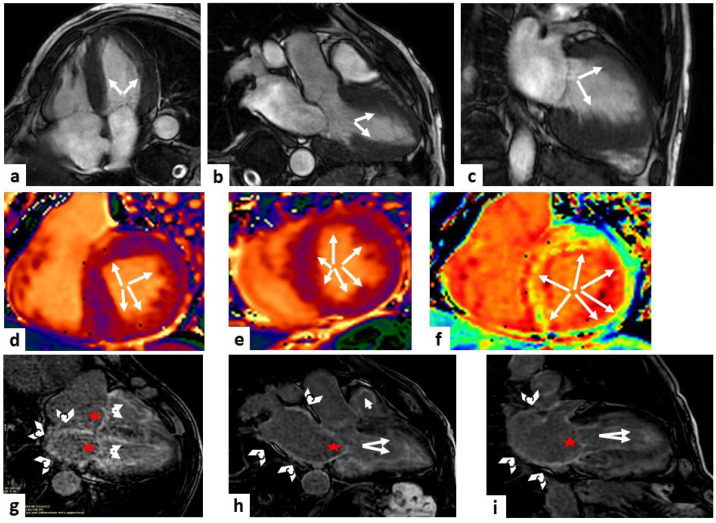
Cardiac amyloidosis. A case of a 79-year-old man, with magnetic resonance imaging (MRI) showing a hypertrophic (white arrows) left ventricle (LV) of 17 mm in the steady-state free precession (SSFP) sequences (panels (a–c) show four-chamber, three-chamber, and two-chamber views, respectively). Mid panels show diffuse high values at T1 mapping sequences, represented by the orange tonality as indicated by the white arrows (panels (d,e) at the level of the basal and mid-LV short-axis views, respectively) and diffuse high values at extracellular volume (ECV) sequence, represented by the yellow tonality as indicated by the white arrows (panel (f) at the level of the basal LV short-axis view). Late gadolinium enhancement (LGE) sequences showed a non-ischemic enhancement pattern in both ventricles (white arrows), a hyperenhancement of the atrio-ventricular valves (red stars), of the left atrium wall and the interatrial septum (white arrowheads) (panels (g–i) show four-chamber, three-chamber, and two-chamber views, respectively). All these findings are diagnostic of cardiac amyloidosis.
